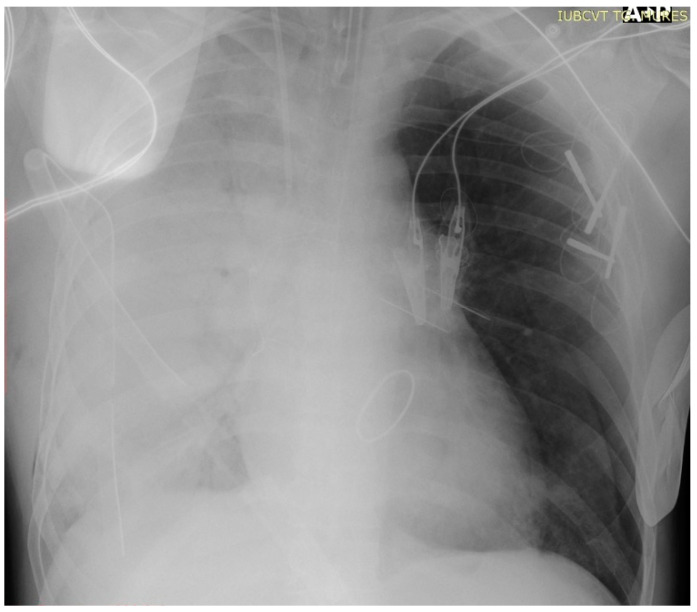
Thoracic X-ray showing right unilateral pulmonary edema.
